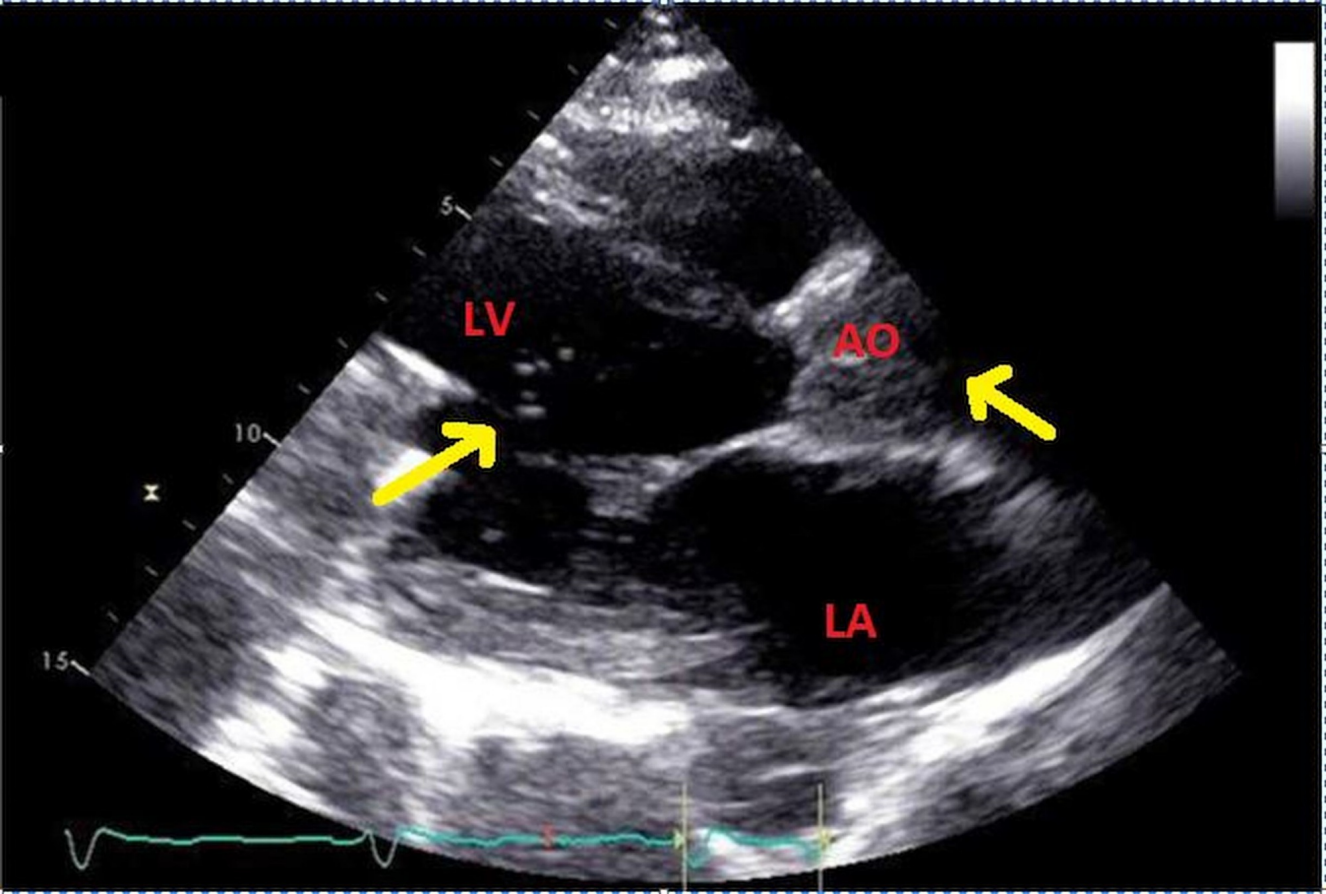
Transthoracic echocardiogram showed microbubbles (arrows) in the aorta and left ventricle LV: left ventricle; AO: aorta; LA: left atrium
